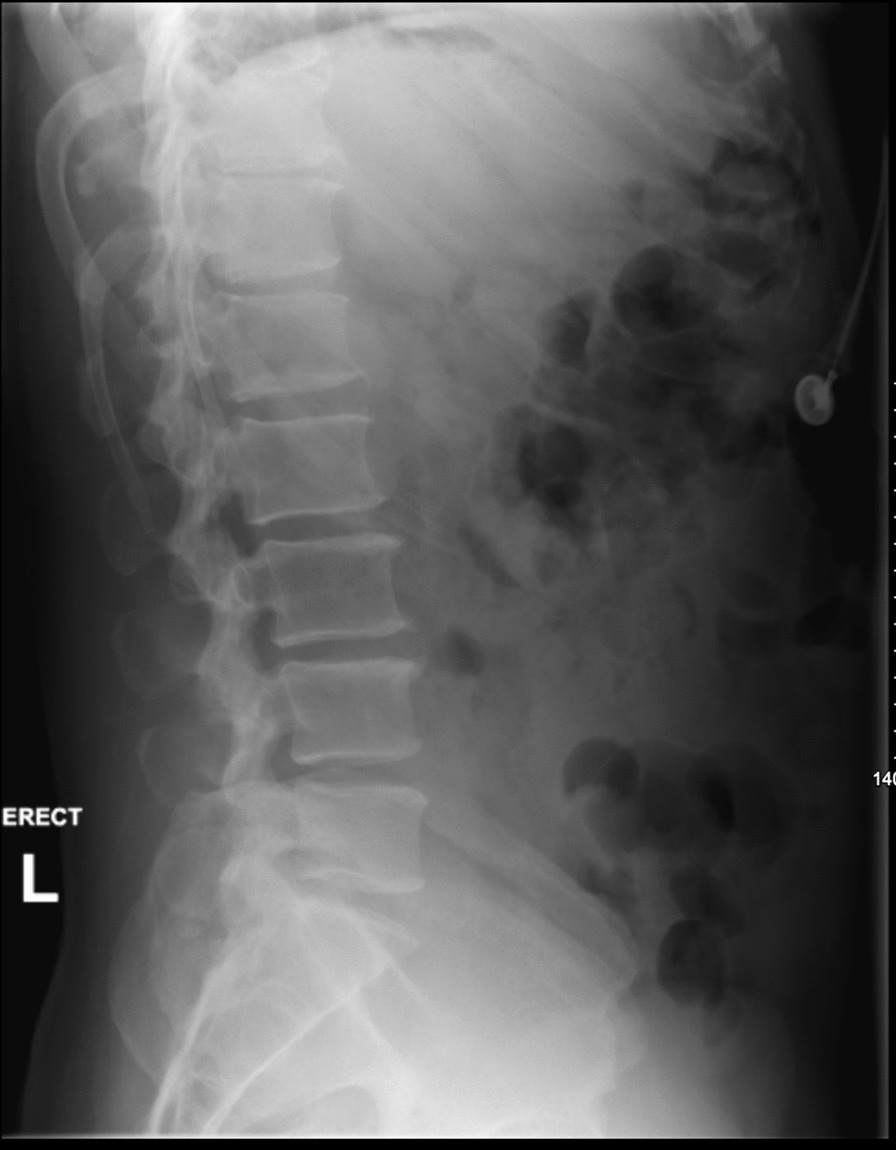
Lateral X-ray image of the lumbar spine demonstrating straightening of the lumbar lordosis, otherwise no fractures or dislocations
